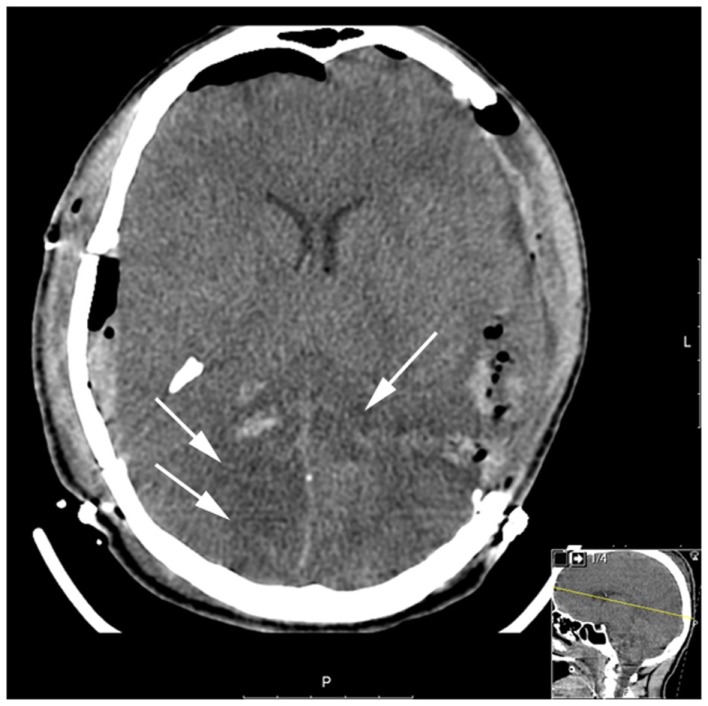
Post-operative CT (after hemicraniectomy) day 2. The white arrows highlight the hypodense regions of brain parenchyma in the posterior circulation which is tissue at risk for subsequent ischemic injury.
